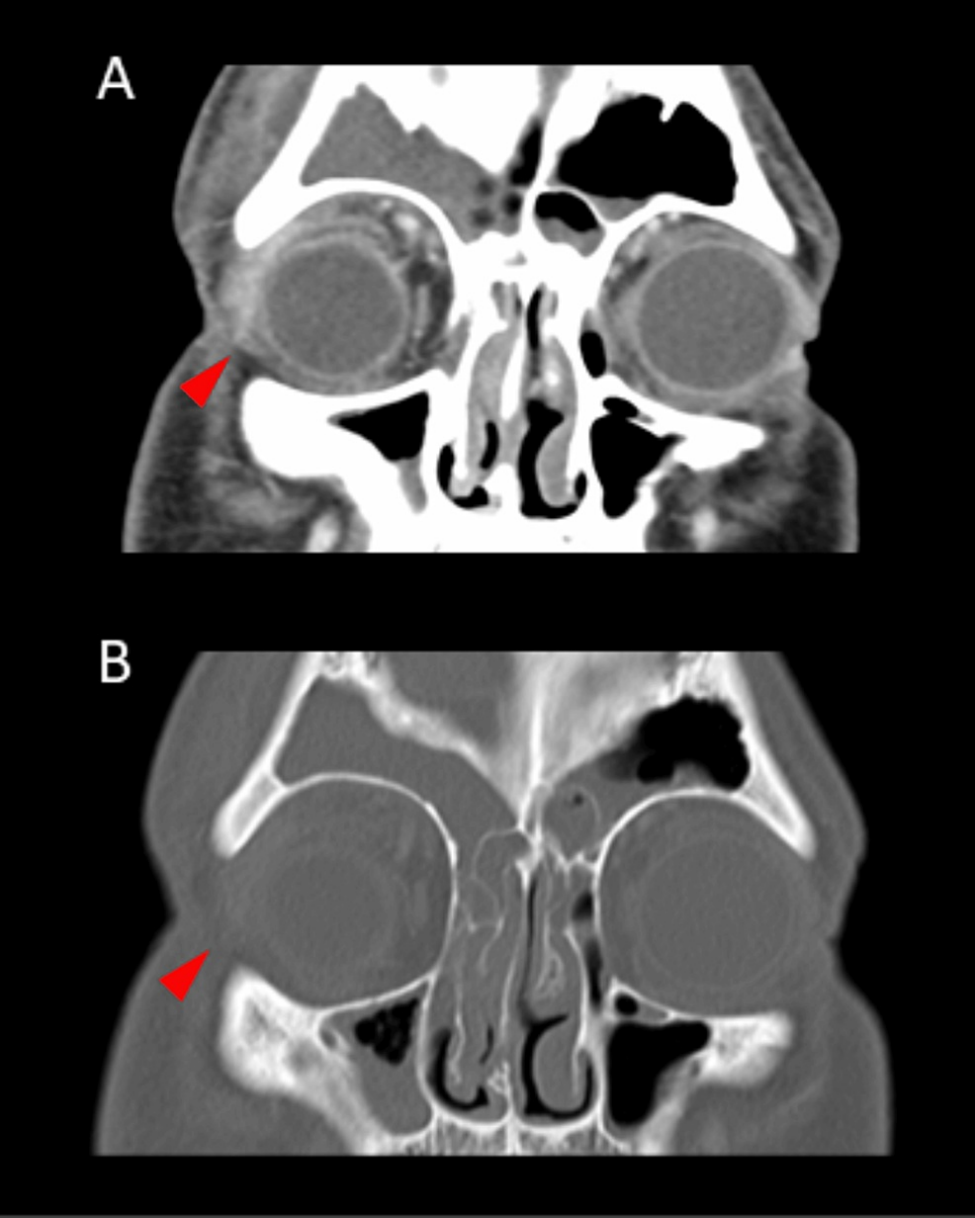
Imaging of the orbital cellulitis A CT scan of the orbits with contrast (A, B) shows right orbital soft tissue swelling (red arrowheads) and compression of the right eyeball.
